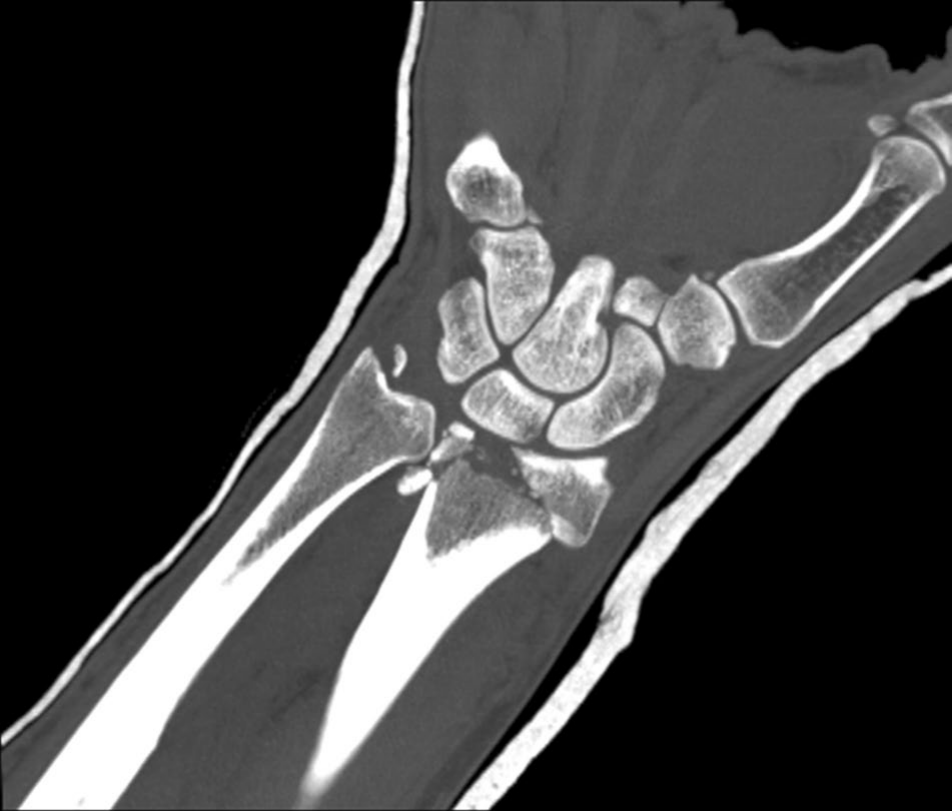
CT scan of a dorsovolar view after reduction and cast immobilization showing the defect zone of the intermediated column and fracture involvement of the DRUJ
